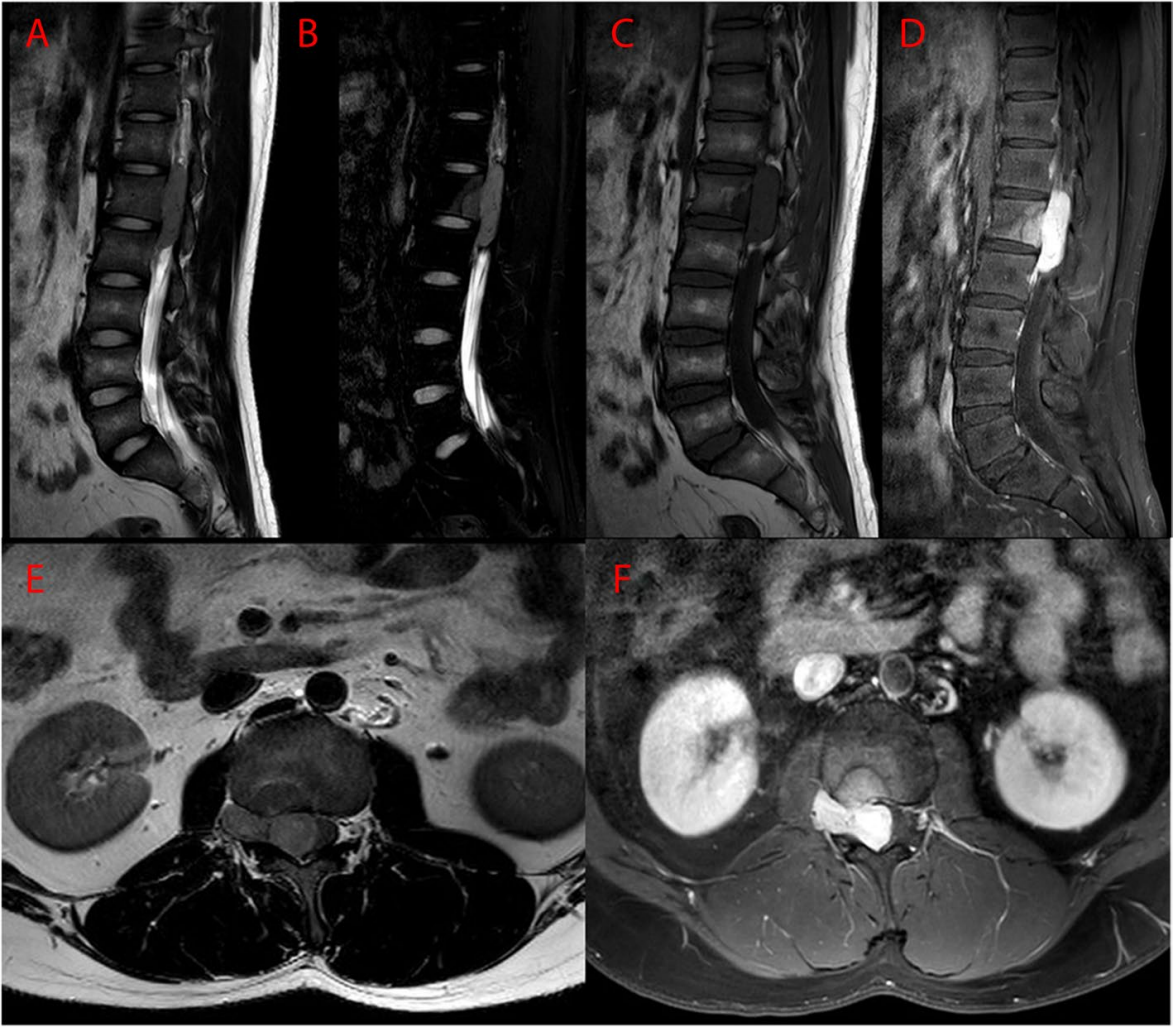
Preoperative MR images. A Sagittal T2WI, B Sagittal T2WI with fat suppression, C Sagittal T1WI, D Sagittal T1WI with gadolinium enhancement, E Axial T1WI, F Axial T1WI with gadolinium enhancement. Dumbbell tumor could be visualized at the L1 level
